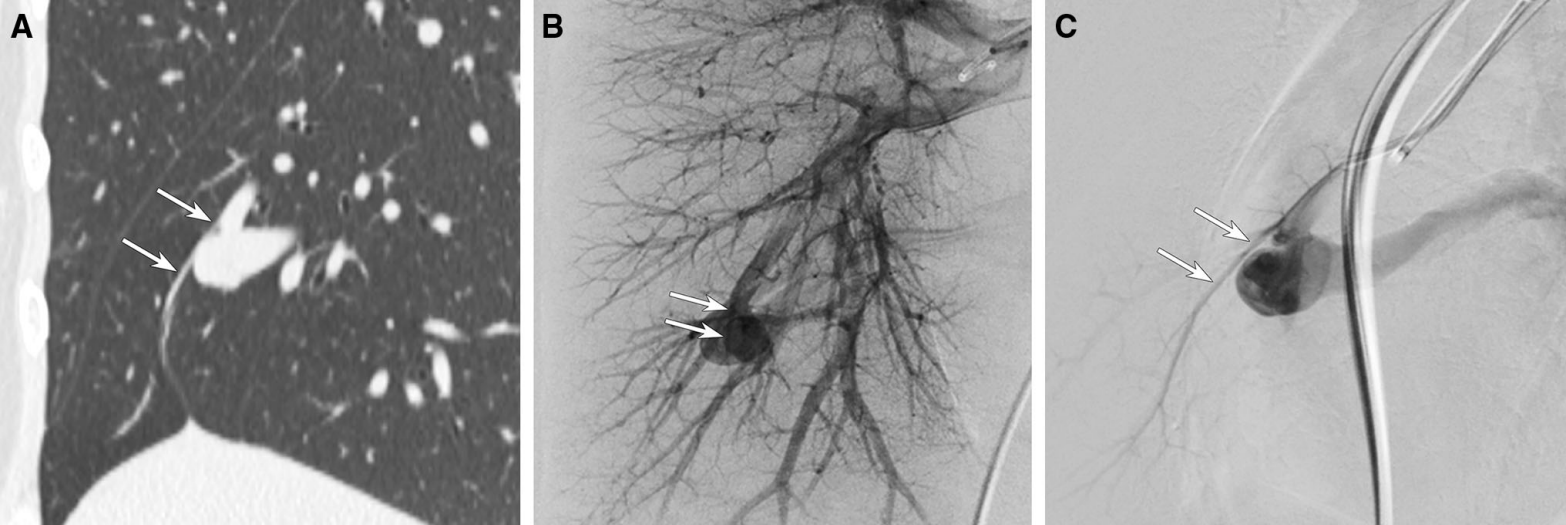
The last normal branch originating from junction visualized on both MDCT and PAG. A Sagittal MPR CT image shows the last normal branch (arrows) originating from junction. B Frontal image of lobar PAG can demonstrate the last normal branch (arrows) from junction detected on MDCT. C Lateral image of selective PAG can demonstrate the last normal branch (arrows)
